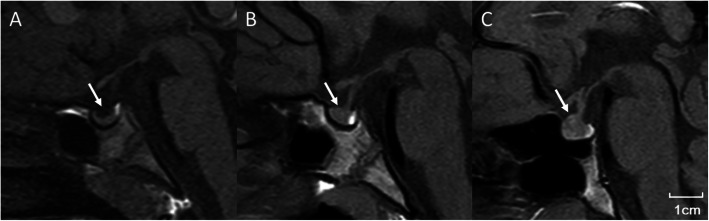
Representative magnetic resonance imaging image for concave (a), flat (b), and convex (c)
